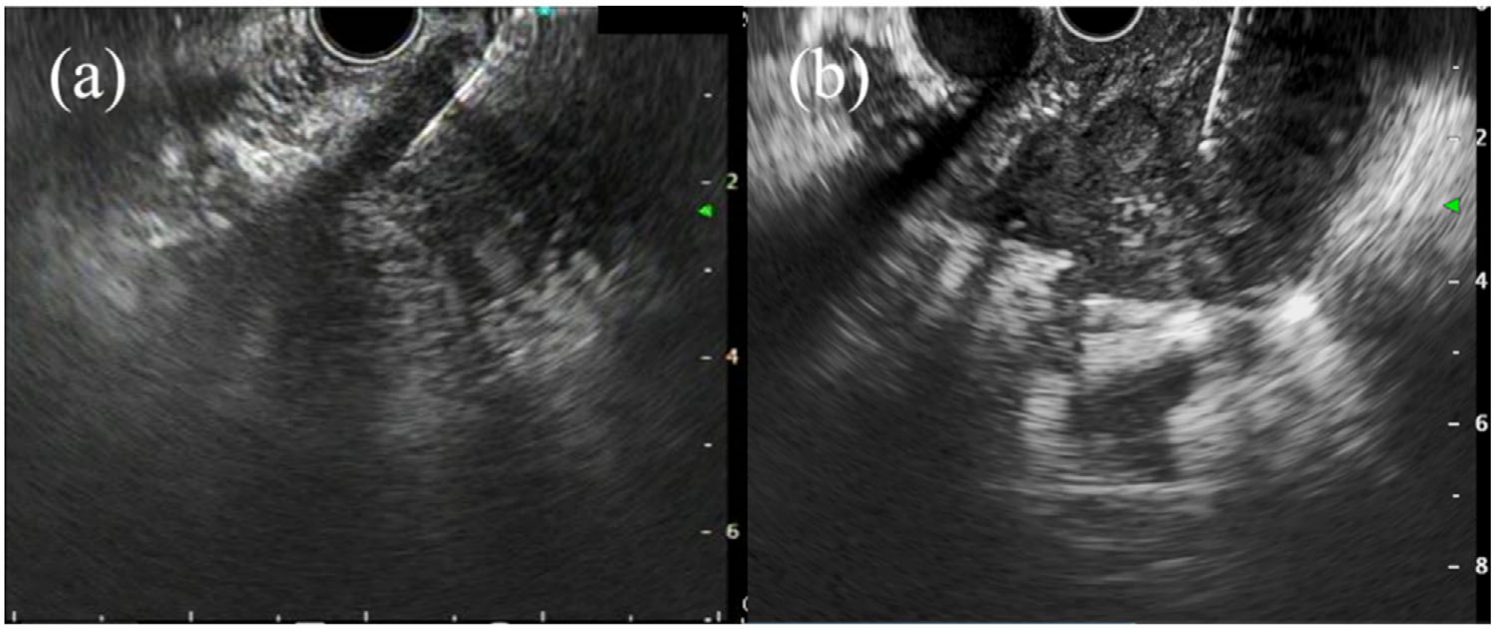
(a) Endoscopic ultrasound‐guided fine‐needle biopsy from the second part of the duodenum using the Franseen needle. (b) Endoscopic ultrasound‐guided fine‐needle biopsy from the second part of the duodenum using the Fork‐tip needle.
